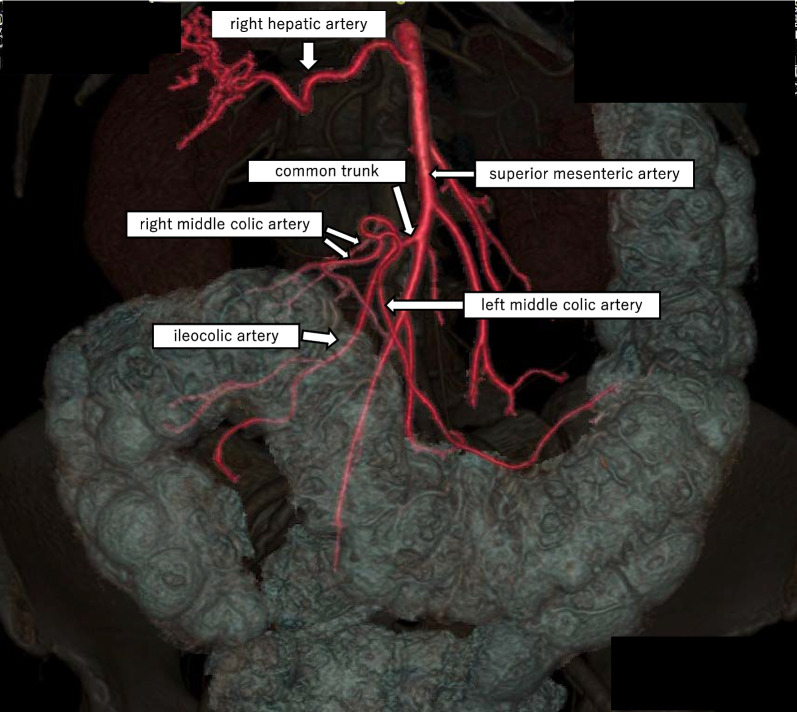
3D-CTA image (artery). 3D-CTA confirmed that the MCA and ICA had a common trunk, and an absent of the RCA
